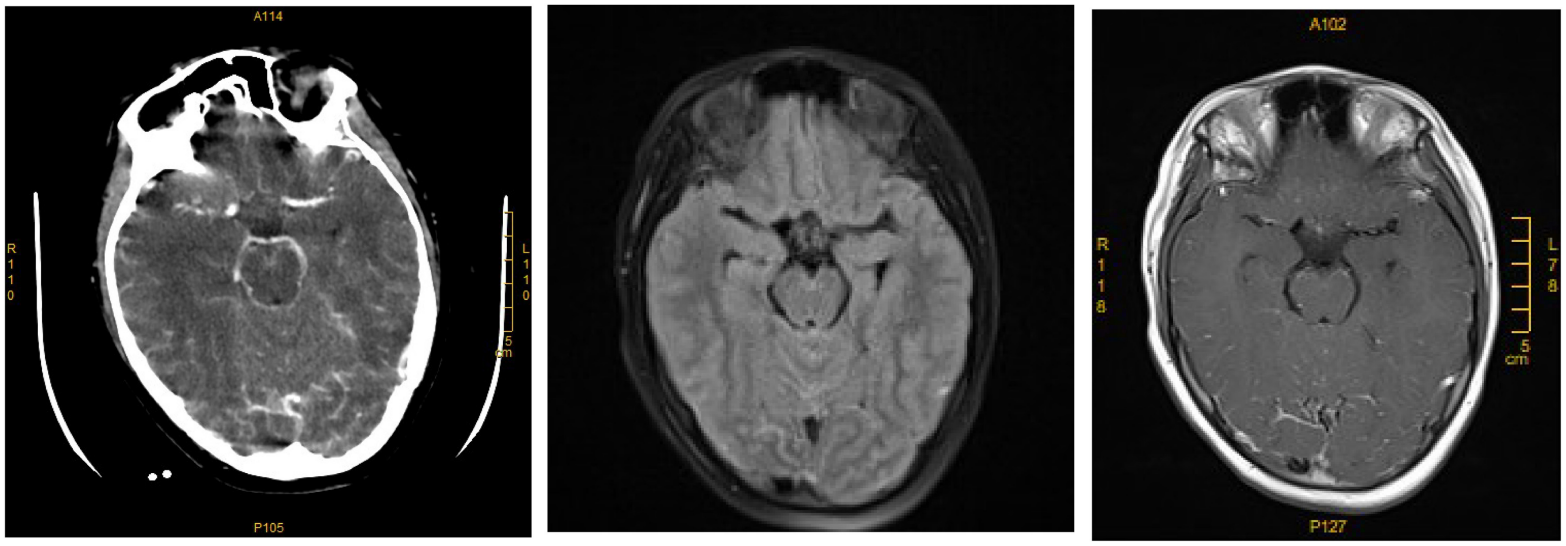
Computed tomography scan and magnetic resonance imaging (MRI) of the primary amoebic meningoencephalitis patient's brain. CT enhancement, T2 flair, and T1 enhancement with leptomeningeal linear enhancement in the occipital lobe.
